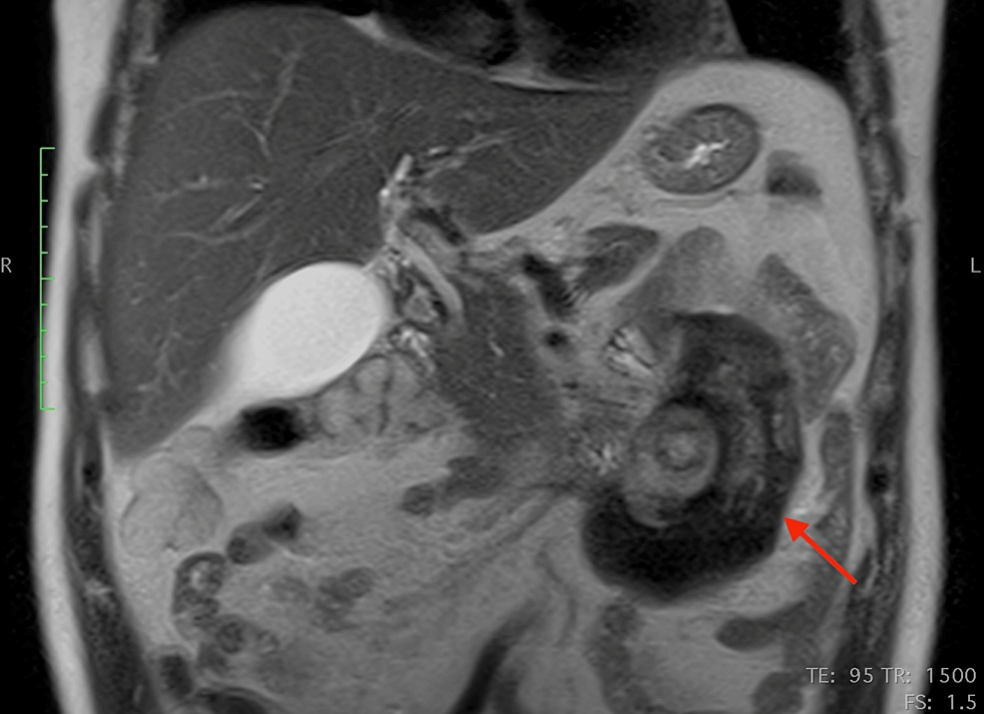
Coronal MRI T2-weighted image (different level compared to Figure 6) The concentric circles-like pattern (like “onion layers”) is best appreciated in the T2 images MRI: magnetic resonance imaging
